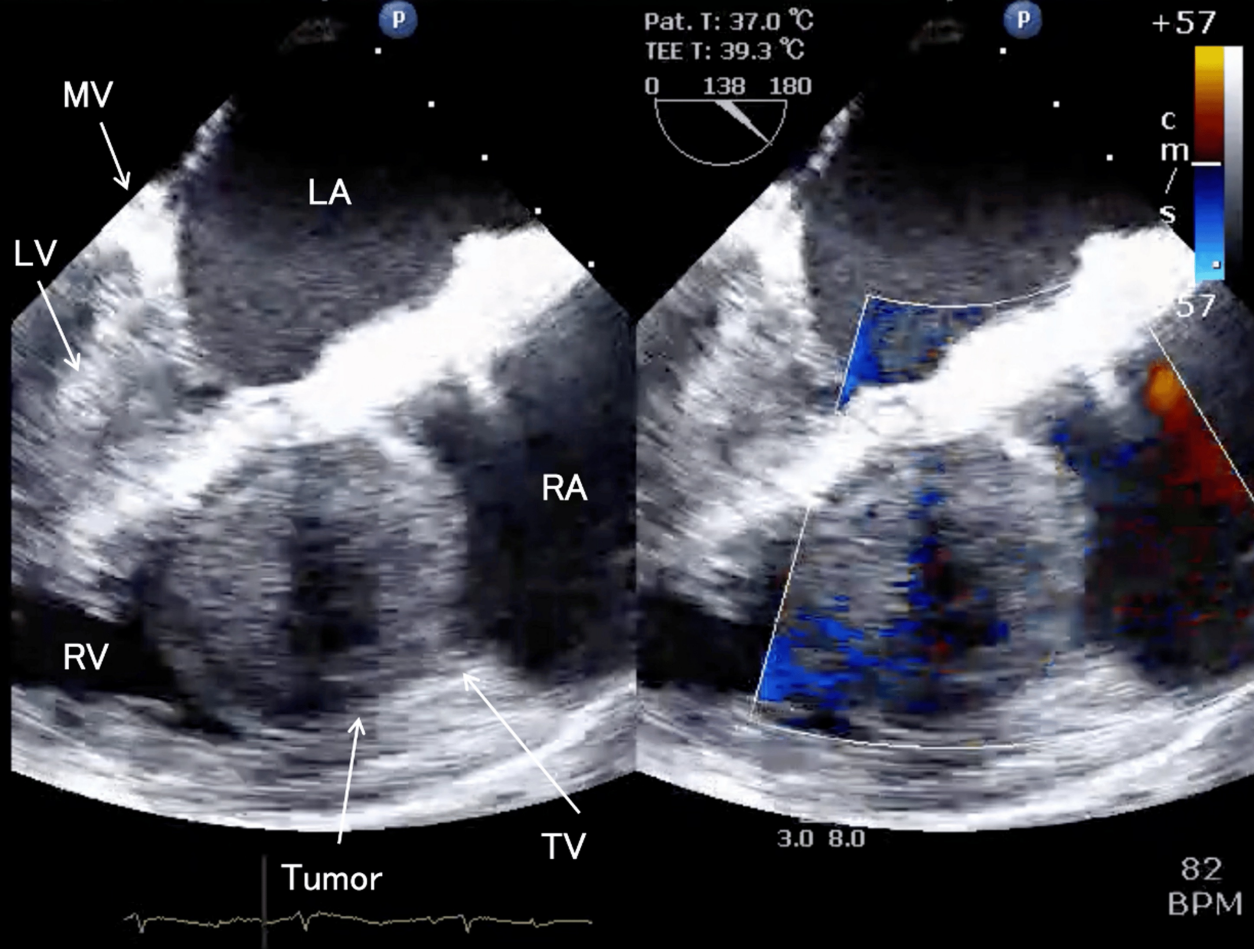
Intraoperative transesophageal echocardiography, color comparing mode of rotated mid-esophageal modified four-chamber view As in Figure 4, a giant tumor is entrapped in the tricuspid valve and obstructs right ventricular inflow. TV, tricuspid valve; RA, right atrium; RV, right ventricle; MV, mitral valve; LA, left atrium; LV, left ventricle
